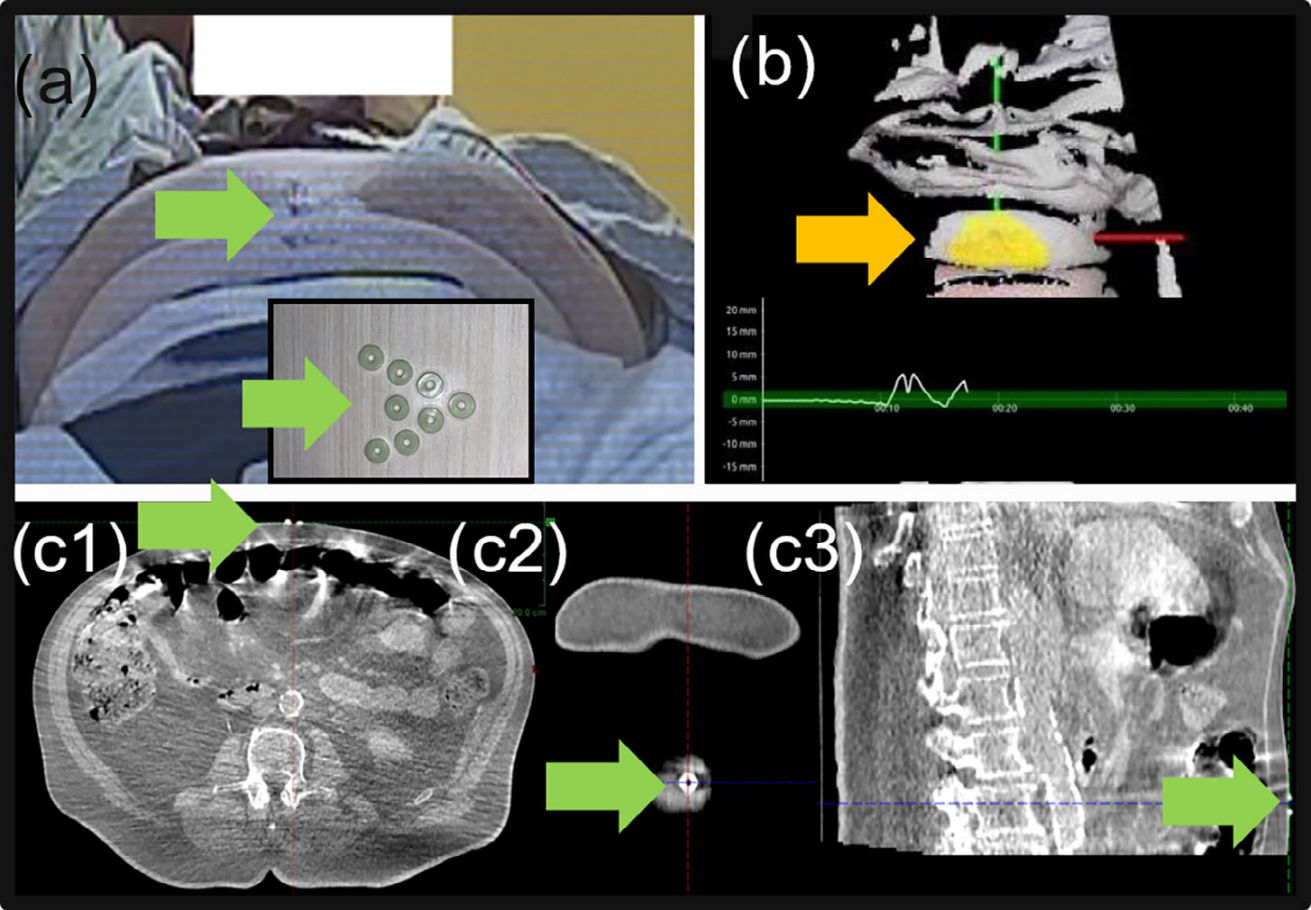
Components of the surface image‐guided Ethos CT‐STAR/SBRT. (a) donut‐shaped imaging‐surrogate on patient's abdomen (green arrow), (b) auxiliary software with real‐time monitoring display (orange arrow for ROI on the abdomen), and (c) donut‐shaped imaging‐surrogate on CBCT. c1: axial, c2: coronal, and c3: sagittal views.
